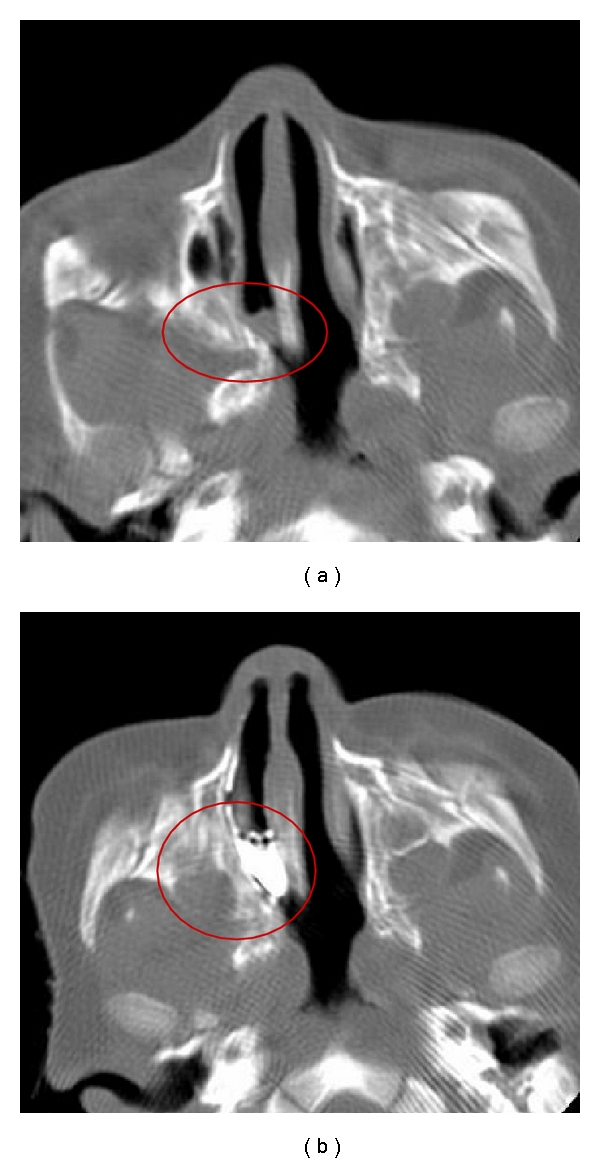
Axial CT before (a) and after (b) local contrast installation in a mixed-type patient. The membrane is thin, not as seen in plain view.
